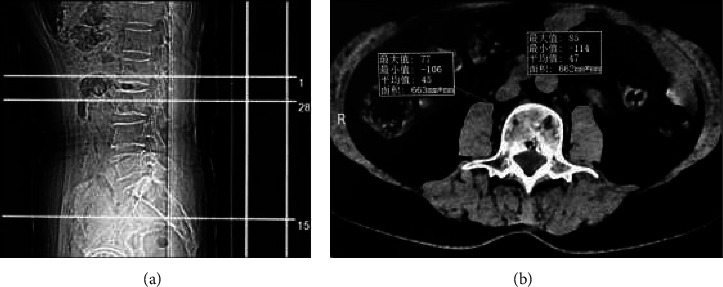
The two images are the CT scan localization image and the CT axial image of a 58-year-old woman with a height of 1.6 m, respectively. (a) The localization image showed that plane of the line 28 was the middle level of the L3 vertebral body. (b) One radiologist manually delineated the edge of the bilateral psoas muscle on the CT axial image of the L3 level. The bilateral psoas muscle area was calculated automatically by the software. The right psoas muscle area was 663 mm^2^, and the left psoas muscle area was 662 mm^2^. According to the calculation formula, the PMI was equal to 517.6 mm^2^/m^2^.
